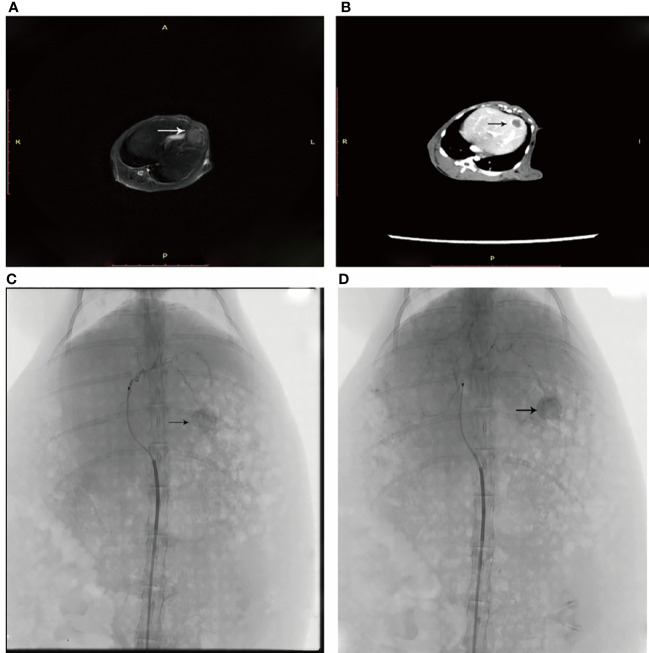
(A) An arrow shows the left lobe of the liver where the neoplasm is in T2 sequence. (B) Enhanced CT shows the tumor in the left liver leaf. (C) DSA angiography shows abundant blood supply to the tumor. (D) An iodized oil deposit is shown by the arrow.
